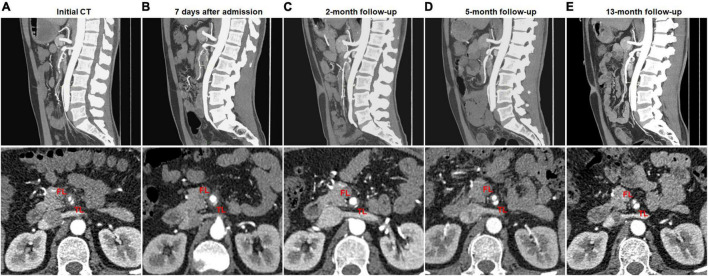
Partial remodeling of type II spontaneous isolated superior mesenteric artery dissection on follow-up computed tomography (CT) angiography. (A) Initial CT angiography showed the patent “cul-de-sac” shaped false lumen without re-entry in a 43-year-old man. (B,C) One week and 2 months after conservative treatment, the false lumen was dilated and severely compress the true lumen. (D,E) Five and 13 months later, the false lumen was progressively reduced, and the true lumen was partially recanalized.
